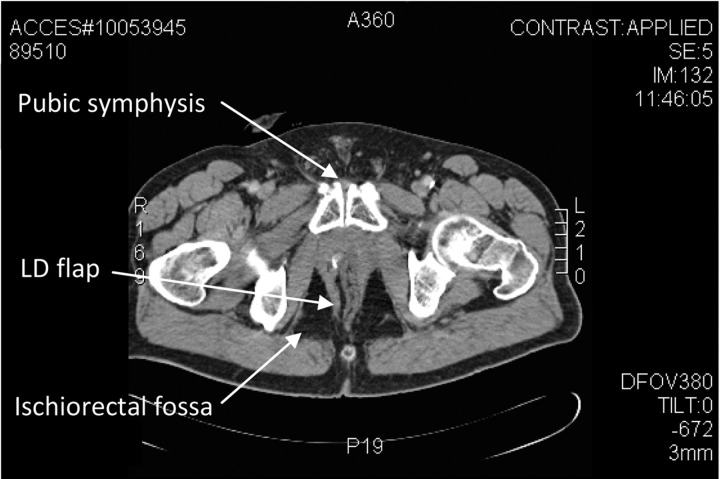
Axial computed tomography of the pelvis following latissimus dorsi (LD) reconstruction of perineum and pelvis
